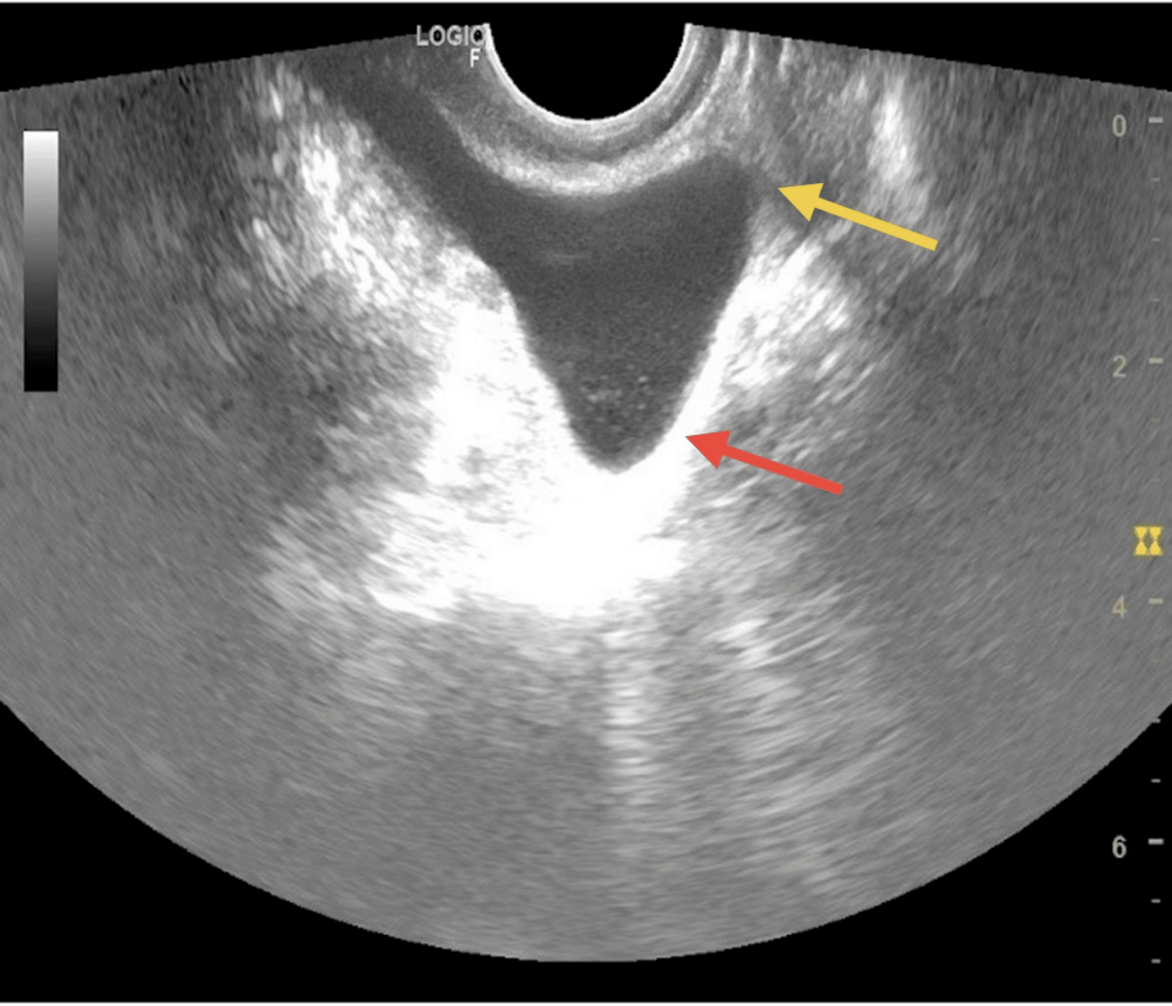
SIS showing unicornuate uterus SIS: saline infusion sonography Yellow arrow showing absence of cornua with tube unilaterally; Red arrow showing unicornuate uterine contour
